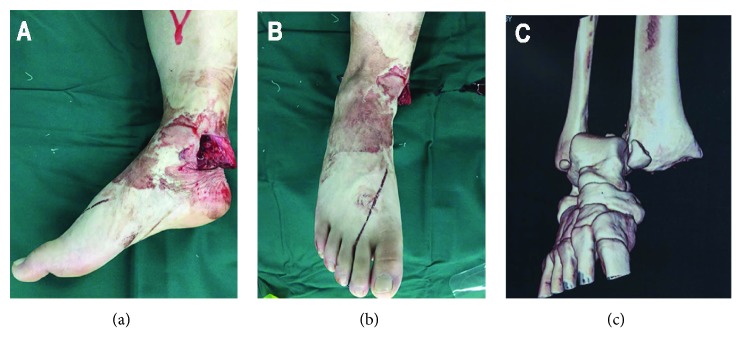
Prereduction view and CT 3D reconstruction of the ankle fracture. (a) Lateral view of the injured ankle. Note displaced distal tibia. (b) Frontal view. (c) CT 3D reconstruction. Talus “wedging” into the distal tibiofibular joint and displacement of tibia are evident.
